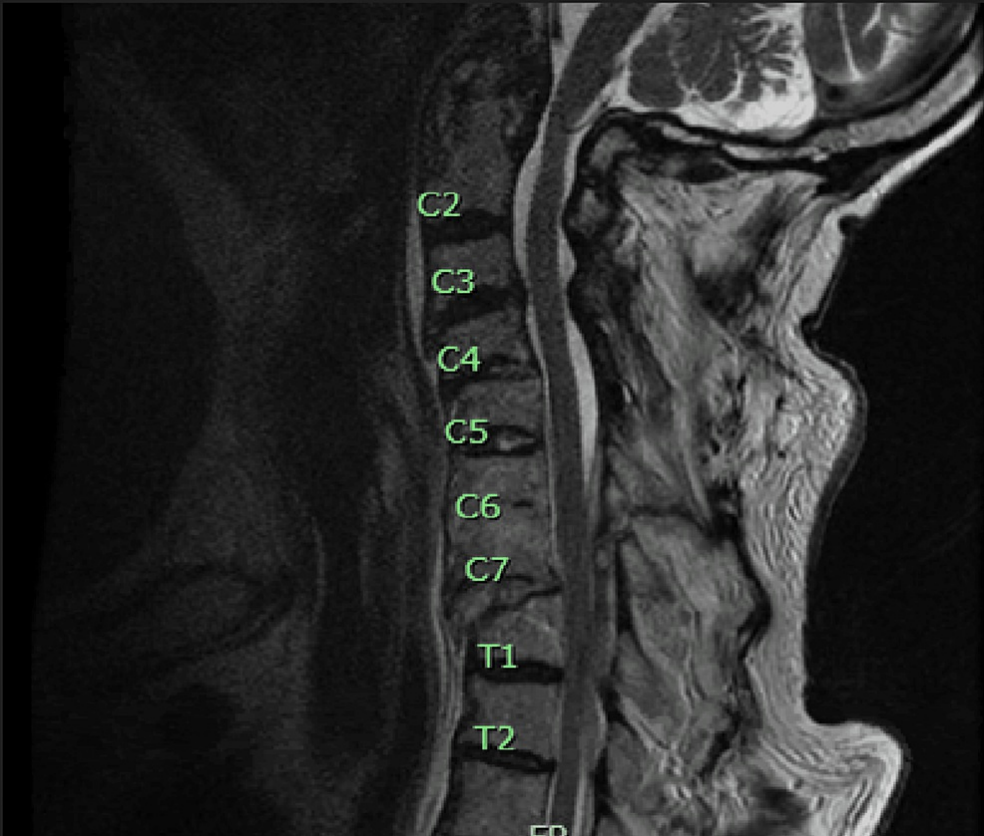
Sagittal T2-weighted MRI demonstrating an acute compression fracture of the superior endplate of T1 vertebra with surrounding edema, an unstable C7-T1 fracture with anterolisthesis, and a dorsal epidural hematoma from C5 to T2.
